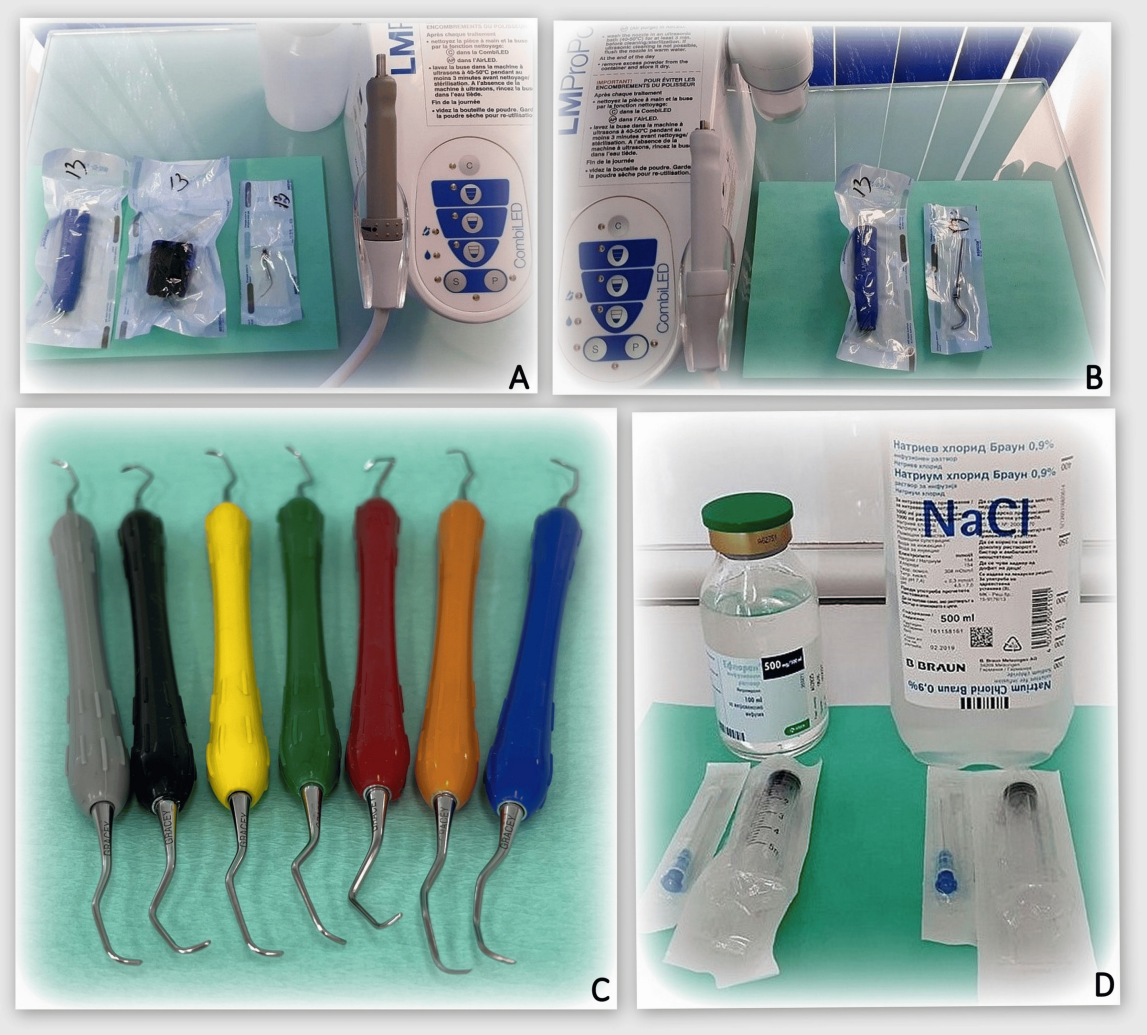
Tools and supplies for performing non-surgical treatment. A: LM-ProPower™ ultrasound device (LM-Dental). B: LM-ProPower™ airflow device (LM-Dental). C: Set of specific Gracey curettes. D: Metronidazole (left) and saline (right).
